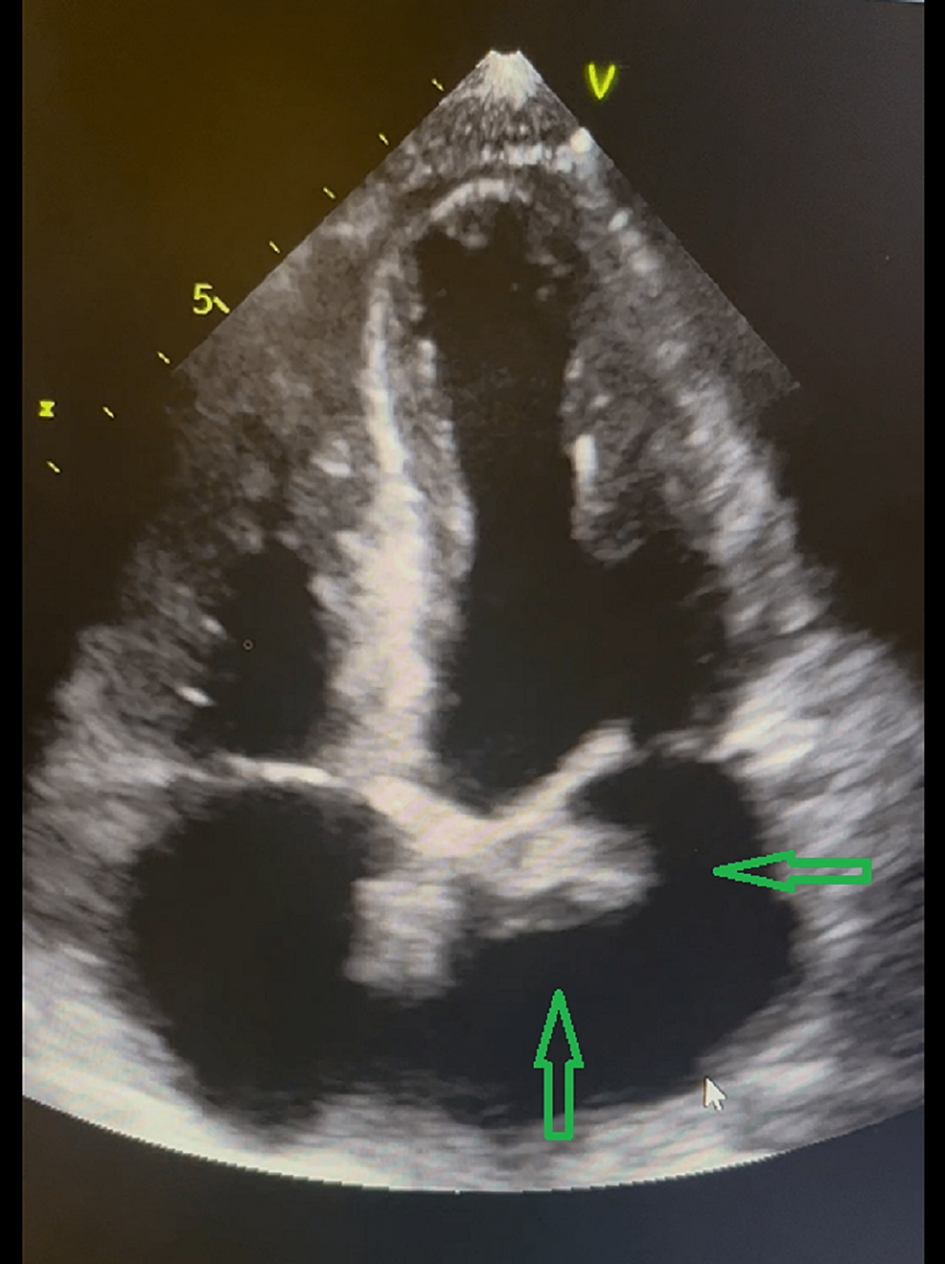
Transthoracic echocardiography four-chamber view showing 1.9x1.7 cm mitral valve vegetation as shown with green arrows
